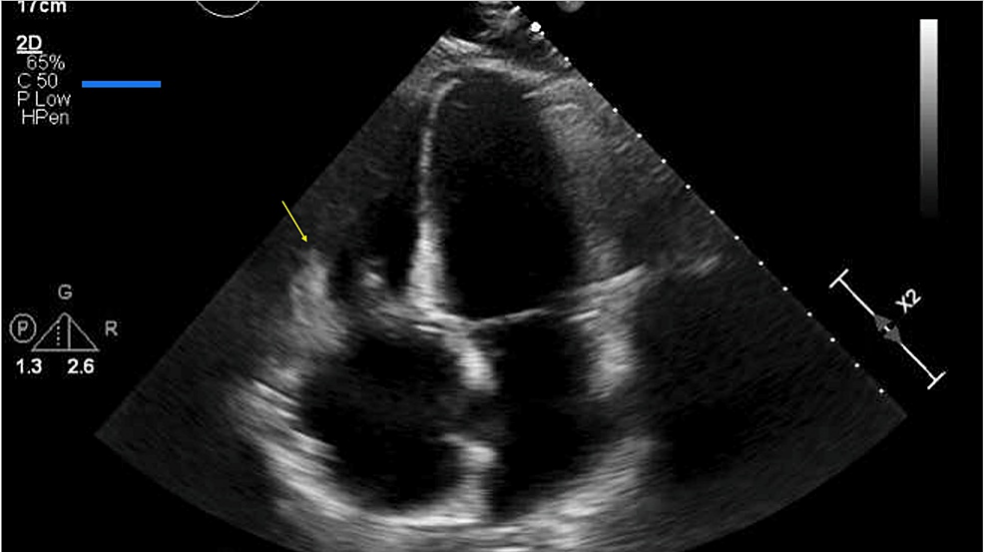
Apical four-chamber view on transthoracic echocardiogram The image is showing medium-sized vegetation on the tricuspid valve.
